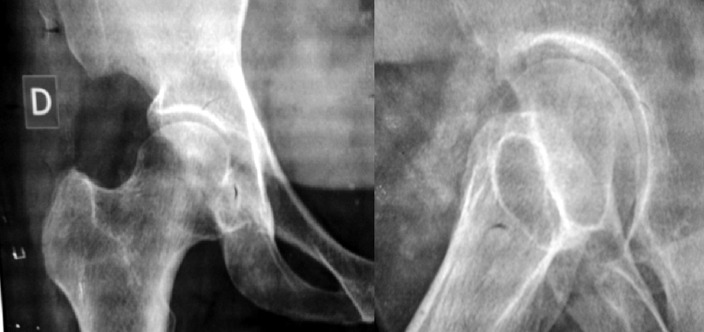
radiographies standards hanche droite face et profil montrant une formation dense sur le versant antérieur du col du fémur
